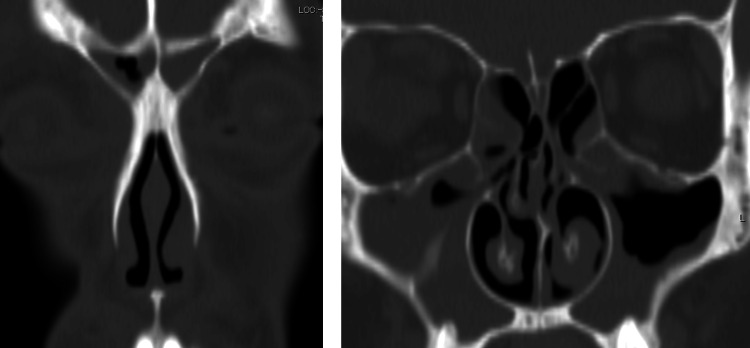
Coronal Sinus CT Coronal section of CT showing soft shadows in the bilateral frontal, maxillary, and ethmoidal sinuses. CT: computed tomography
